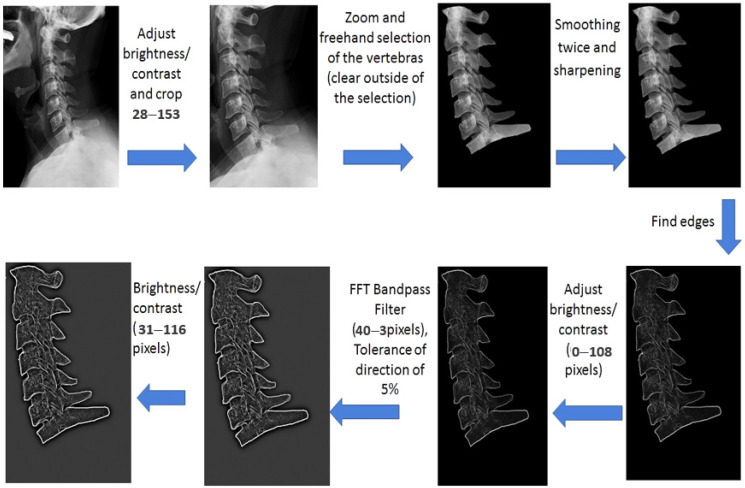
Features extraction using the GLCM method applied to the neck’s X-ray images.
